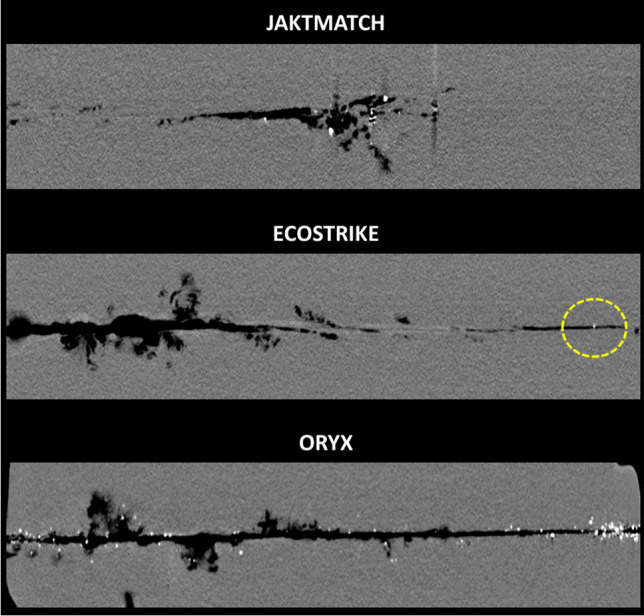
Sagittal computed tomography slice along the bullet channel. Bullet direction was from left to right. Dashed circle is used to demarkate the only fragment identified for Ecostrike
